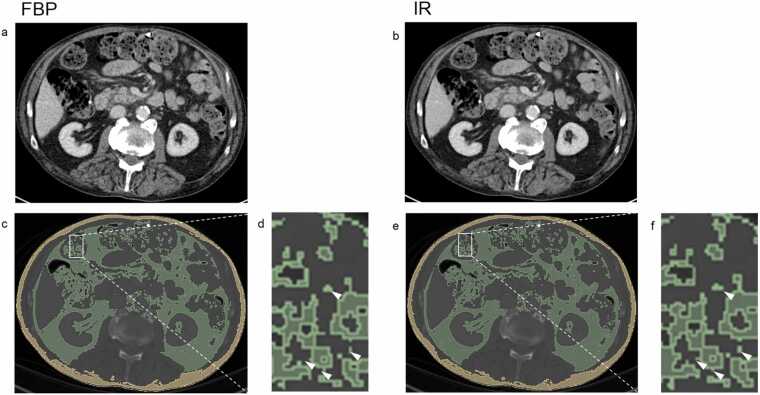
Exemplary CT slice (2D) of the abdomen at the level of L2/3 in venous contrast phase (a, b) and segmented adipose tissue of the same slice (c-f). The CT images on left were reconstructed using filtered back projection (FBP, a,c,d), the CT images on the right were reconstructed using iterative reconstruction (IR, b,e,f). Segmented visceral adipose tissue is marked in green, subcutaneous adipose tissue is marked in yellow. Minor differences in segmentation can be observed between the two reconstruction algorithms (IR and FBP) at tissue interfaces, e.g. in the vicinity of blood vessels or the bowel (arrowheads in d and f). FBP=filtered back projection; IR=iterative reconstruction.
